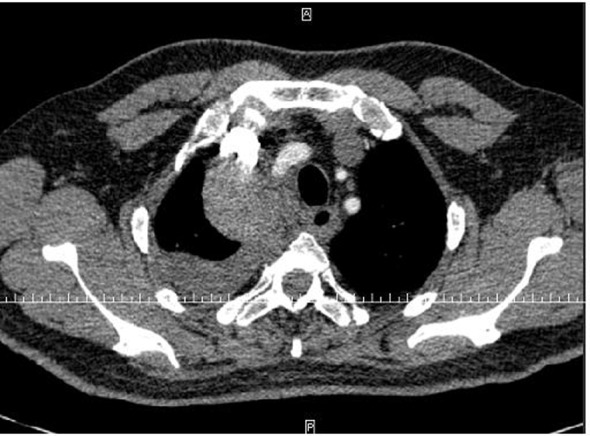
CT angiography showing a loculation in the right upper paramediastinal cervicothoracic region, with active bleeding points dependent on the ascending cervical branch of right subclavian artery.
